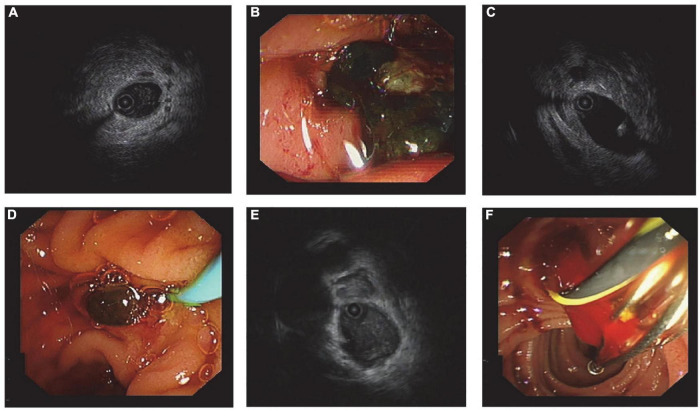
Ultrasonographic and endoscopic images from typical cases. Patient 1: (A) IDUS showed flocculent echo in the lumen; (B) the thick bile was the cause of acoustic characteristics. Patient 2: (C) A little arc high-echo with a shadow was found by IDUS, and it was distinguished from the guidewire, which has ripples in the rear; (D) a small stone with a diameter around 3 mm in size was pulled out. Patient 3: (E) Flocculent echo was detected inside the bile duct, which resembled the acoustic feature of sludge; (F) blood clot was taken out from the common bile duct, and that was because blood stream entered backward into bile duct during sphincterotomy.
